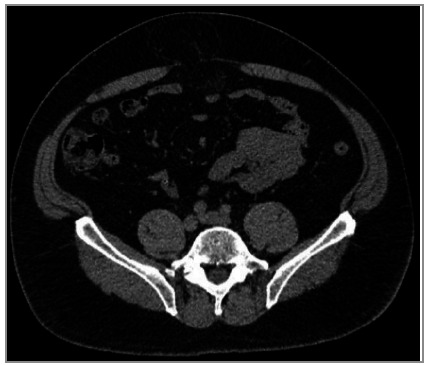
CT image showing a small hernia defect but with important diastasis/weakness of the AW.
